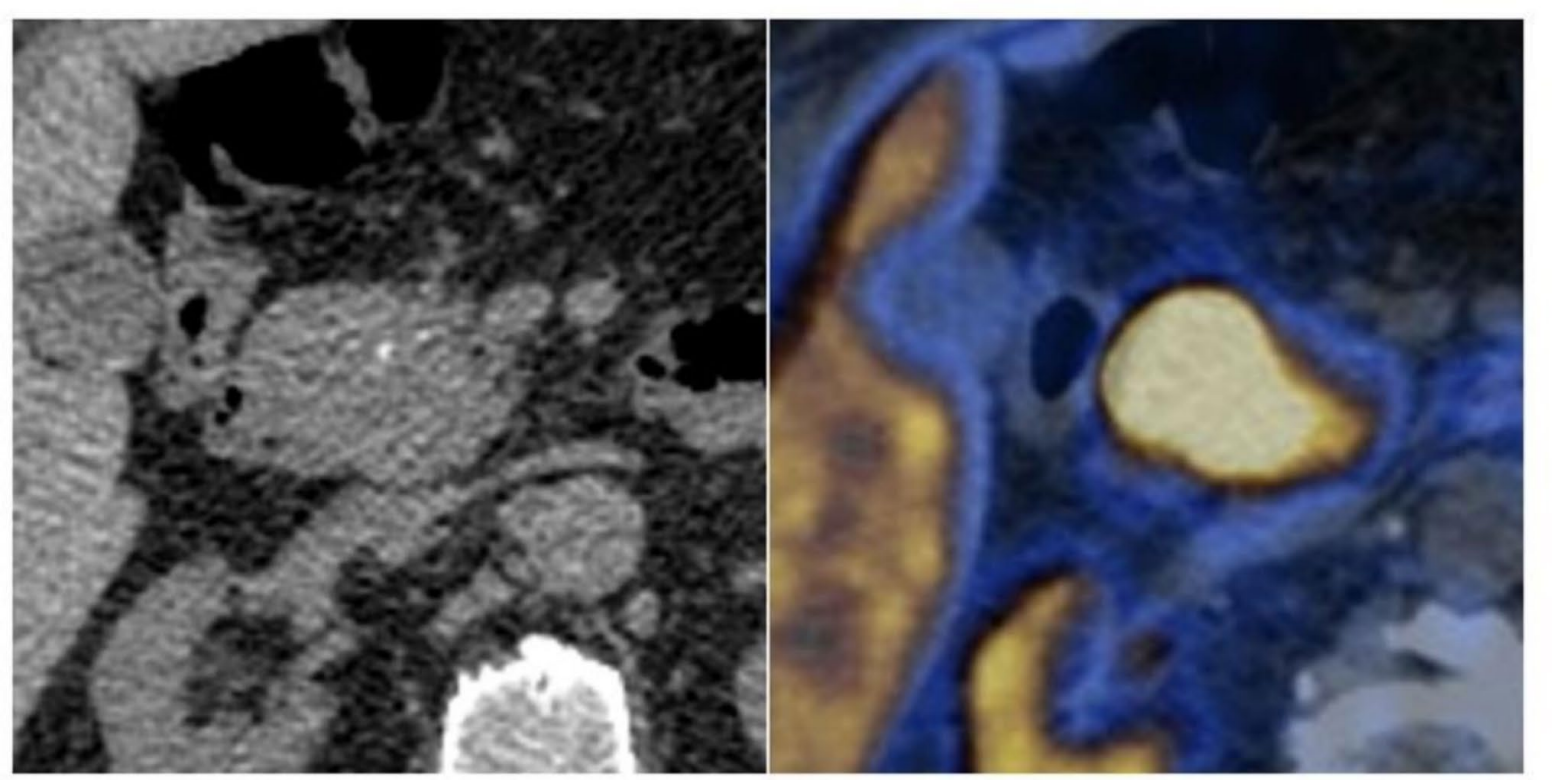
49-year-old man with pancreactic neuroendocrine neoplasm. a CT image showing intratumoral punctate calcifications (type 1 pattern); b at PET-CT scan the lesion intense ^68^Gallium uptake
